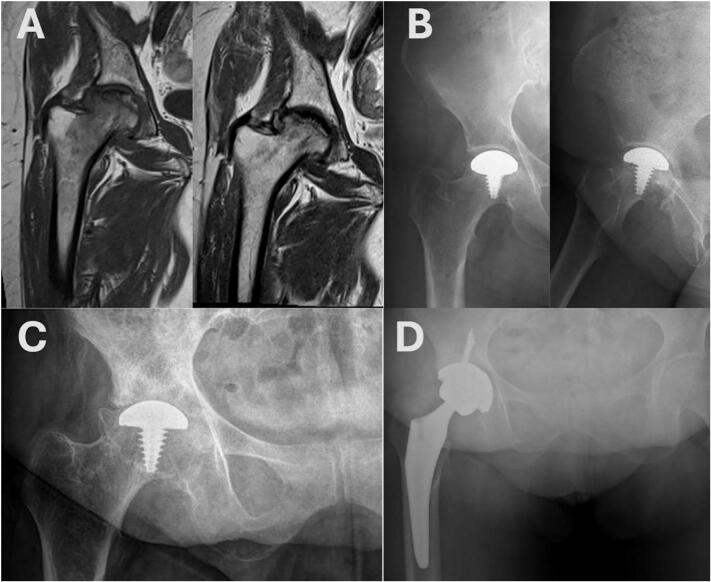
T1-weighted MRI sequences of the right hip obtained, respectively, prior to core decompression and immediately before HemiCAP® resurfacing arthroplasty (drill holes in the femoral neck from the core decompression procedure can be observed). (A) Anteroposterior and lateral hip radiographs of the patient (respectively) at postoperative year 1 following HemiCAP® resurfacing arthroplasty. (B). Preoperative anteroposterior pelvic radiograph of the patient prior to total hip arthroplasty (C). Anteroposterior pelvic radiograph of the patient at postoperative year 1 following HemiCAP® resurfacing arthroplasty (D).
